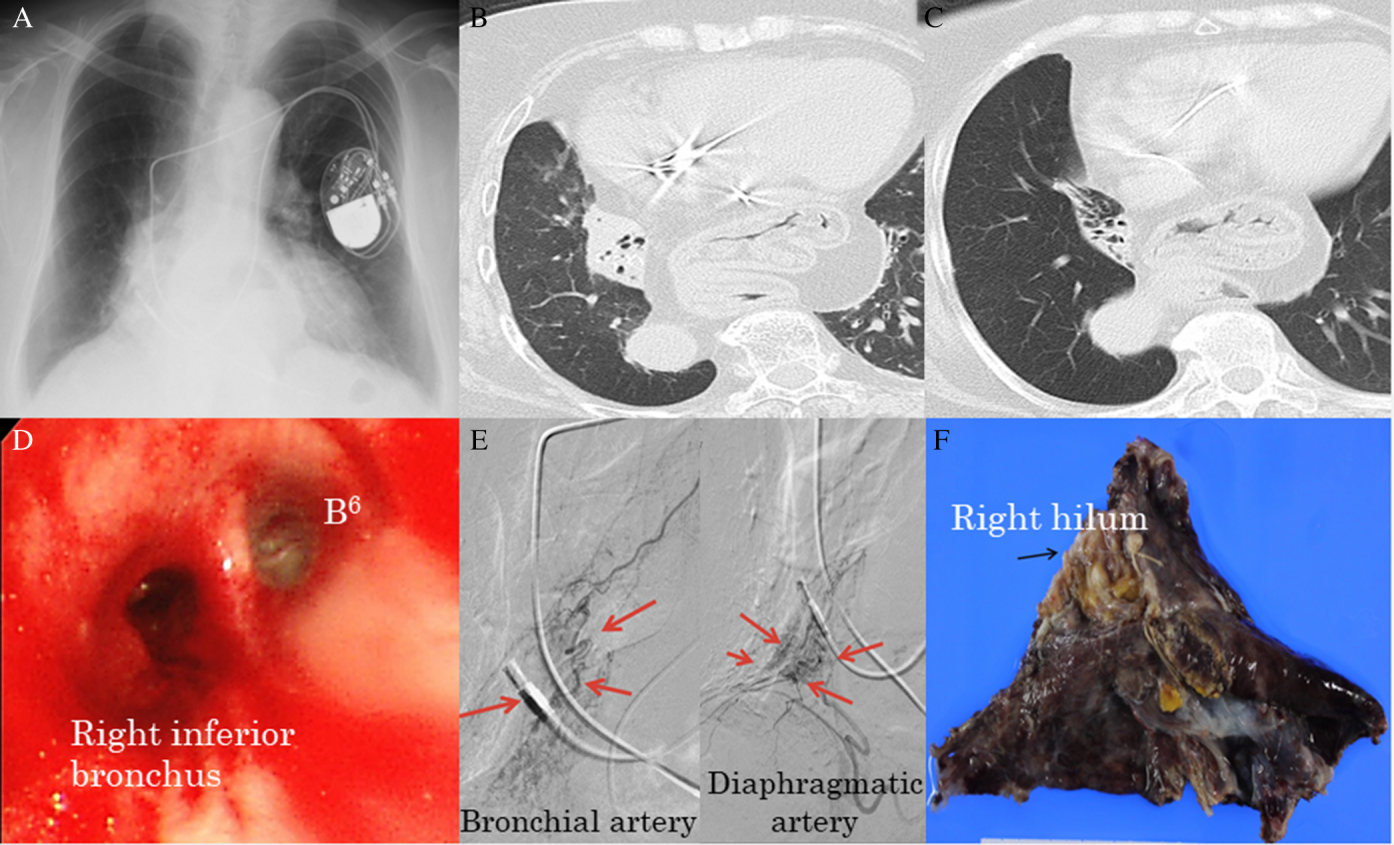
Chest radiograph showed a restiform shadow in the right middle to lower lung field (A). Chest computed tomography (CT) showed a solid nodule in the right lower lobe at the time of admission (B). Chest CT performed five years ago showed atelectasis in the small right lower lobe (C). Bronchoscopy showed the right inferior bronchus completely filled with blood clots (D). The bronchial artery angiography showed the expansion and meandering of the bronchial arteries and the growth of the reticular artery from the diaphragm arteries (E). Macroscopic appearance of the right middle lobe and resected tumour (F).
